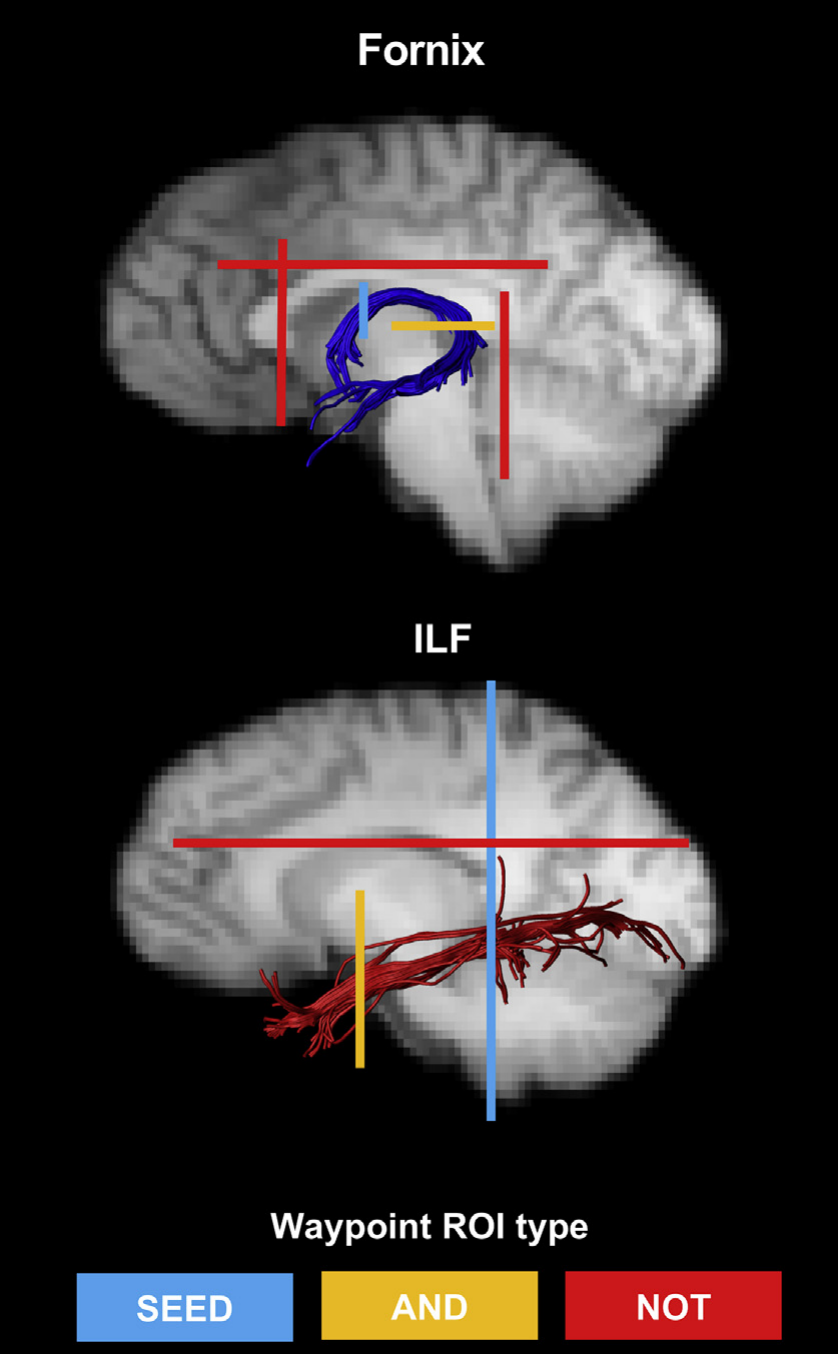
Example reconstructions for the fornix and inferior longitudinal fasciculus (ILF). The waypoint regions-of-interest (ROIs) used for reconstructing each tract are depicted on each image (SEED ROI = Blue; AND ROI = Orange; NOT ROI = Red). The tracts are displayed on sagittal midline slices of a participant's T1-weighted brain image (registered to native diffusion space).
